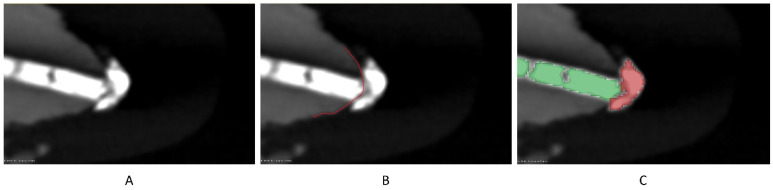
The distinction between the filling inside the canal (green) and the extrusion (red) (C) achieved by following the tooth contour (B) after micro CT scan (A).
